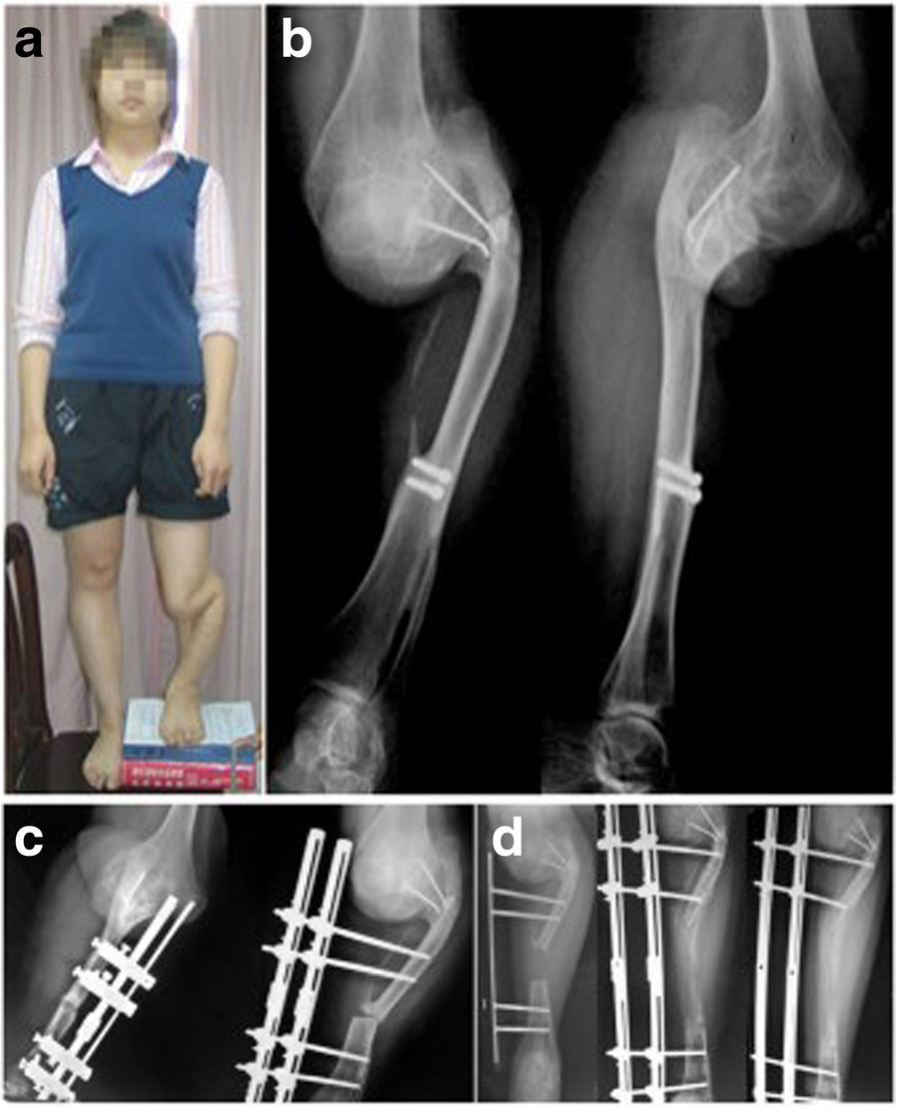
This clinical image of the case 2 shows 9 cm shortness at the left limb (a), and the preoperative X-ray film reveals her upper and most middle left tibia involving the proximal epiphysis were fusioned together with fibula (b), and the length correction program with unilateral external fixator was done and the callus regenerated well (c, d)
